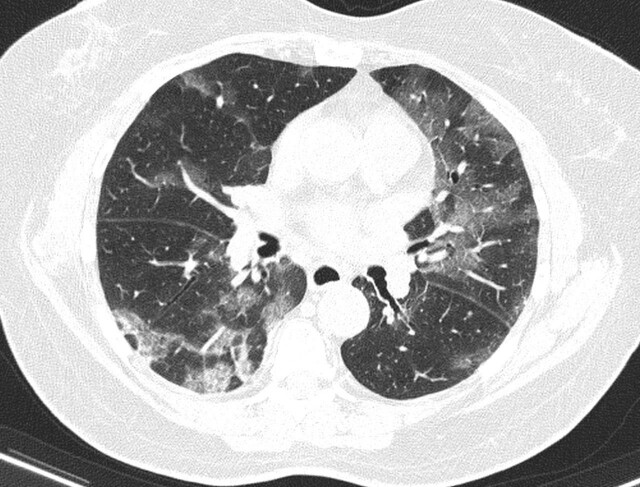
CT of a patient with severe COVID-19. Observation of the bilateral ground glass lesions and interlobular septal thickening, suggestive of COVID-19.
